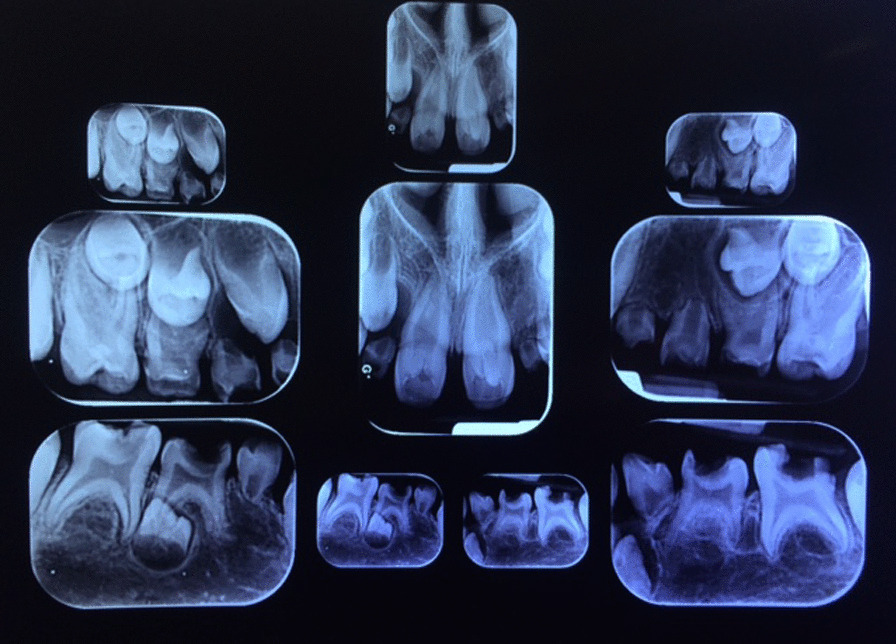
Pretreatment PA radiographs
